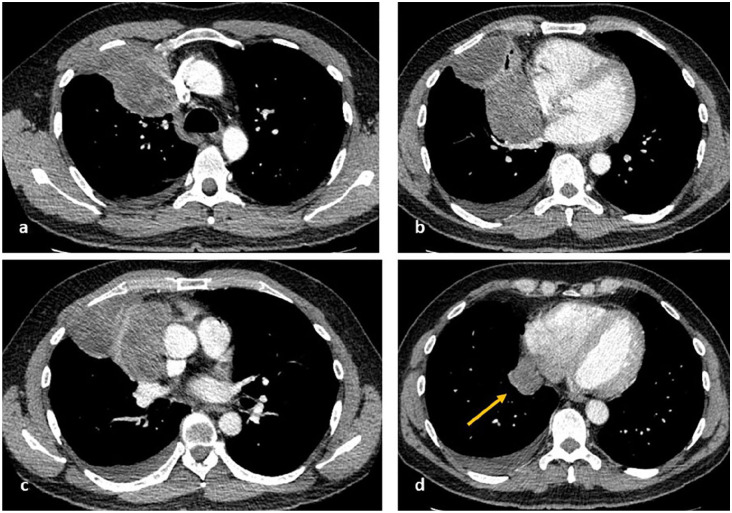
Preoperative computed tomography shows a right mediastinal mass with possible involvement of superior vena cava, right brachiocephalic vein, right main pulmonary artery, right superior and inferior pulmonary veins, and the pericardium surrounding the right atrium inferior to the right hila continuous with right basal para mediastinal pleural deposits (arrow). There is also extensive involvement of the right pleura with right anterior chest wall involvement.
